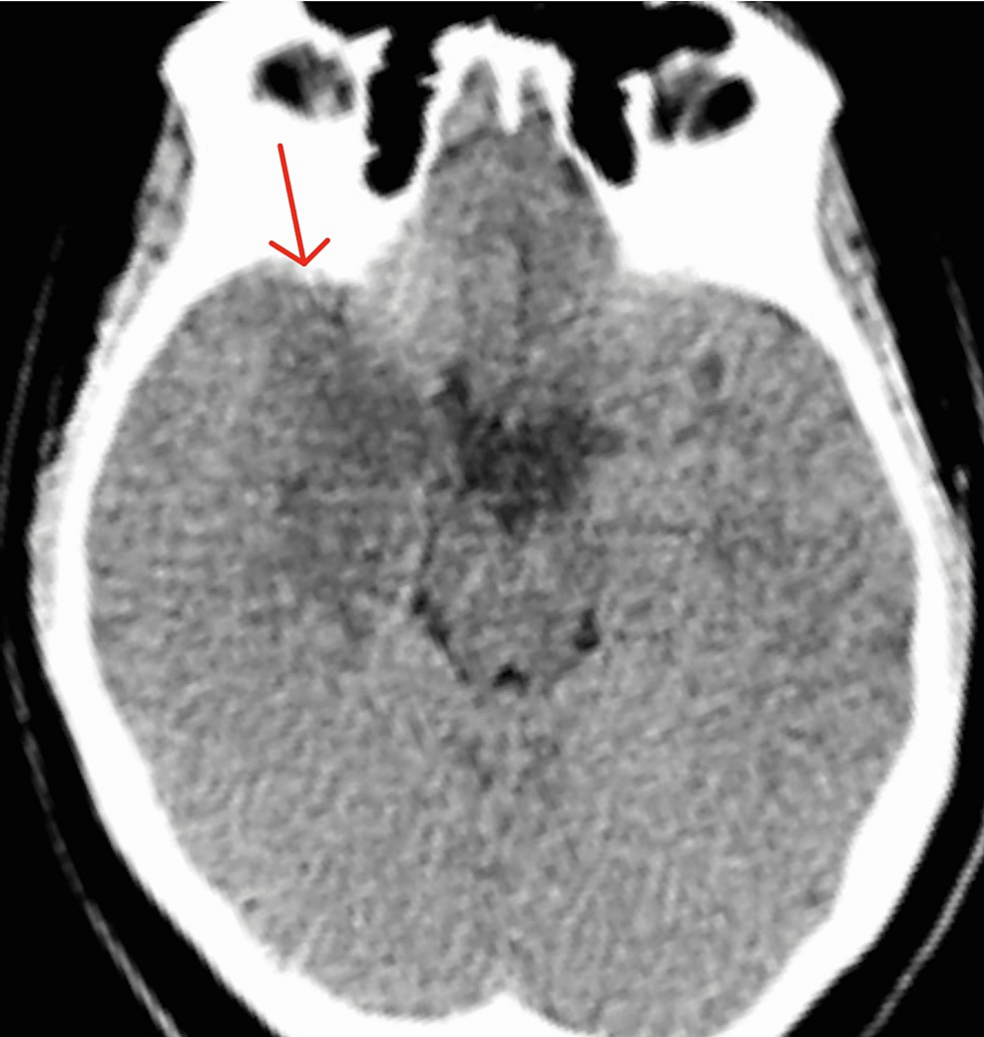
Repeat CT brain without contrast CT brain without contrast shows a new ill-defined hypoattenuation intermixed with bandlike curvilinear hyper attenuation in the right medial temporal lobe (red arrows).
